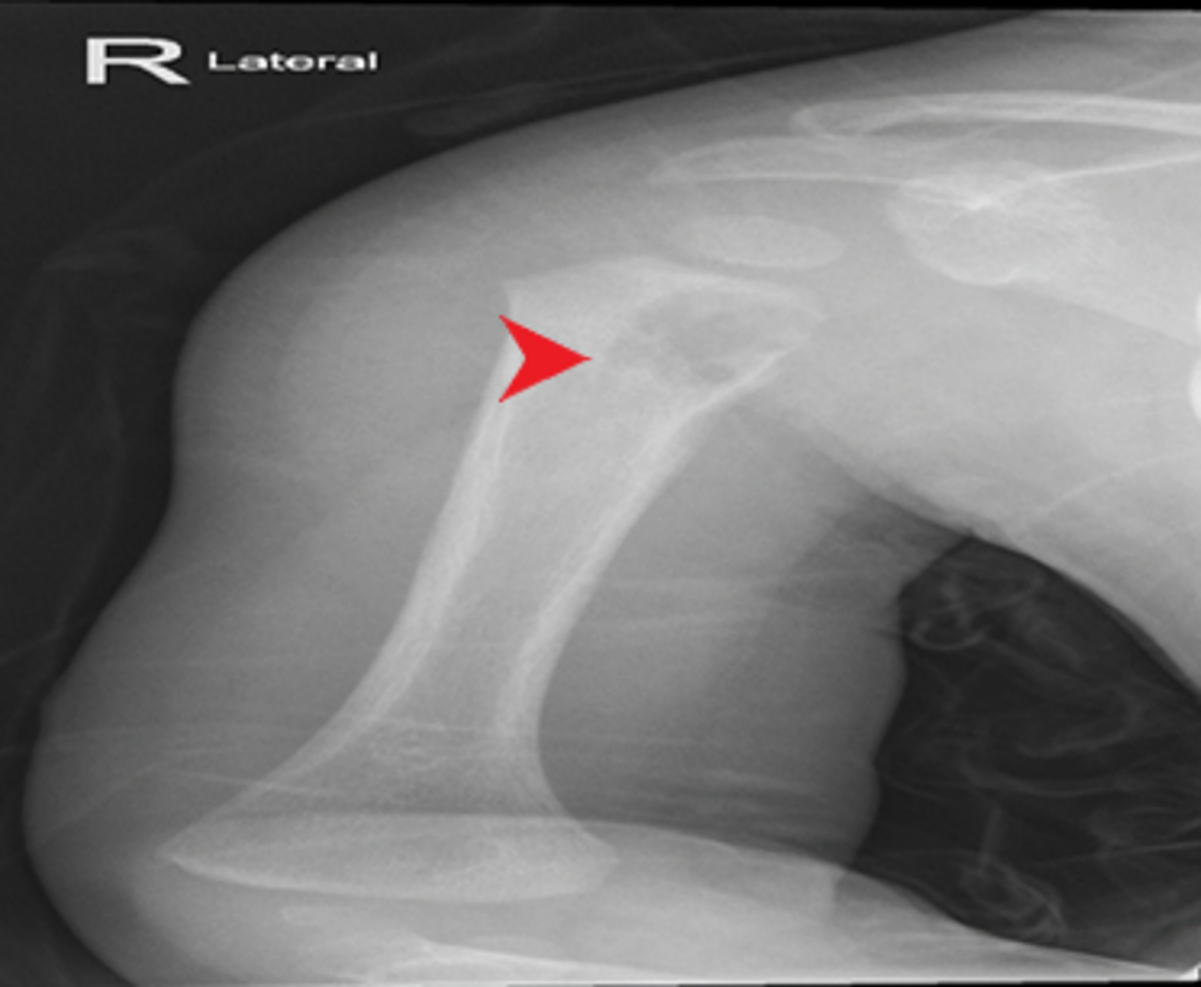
X-ray shows a sharply demarcated lytic lesion with cortical erosion in the right proximal humerus.
